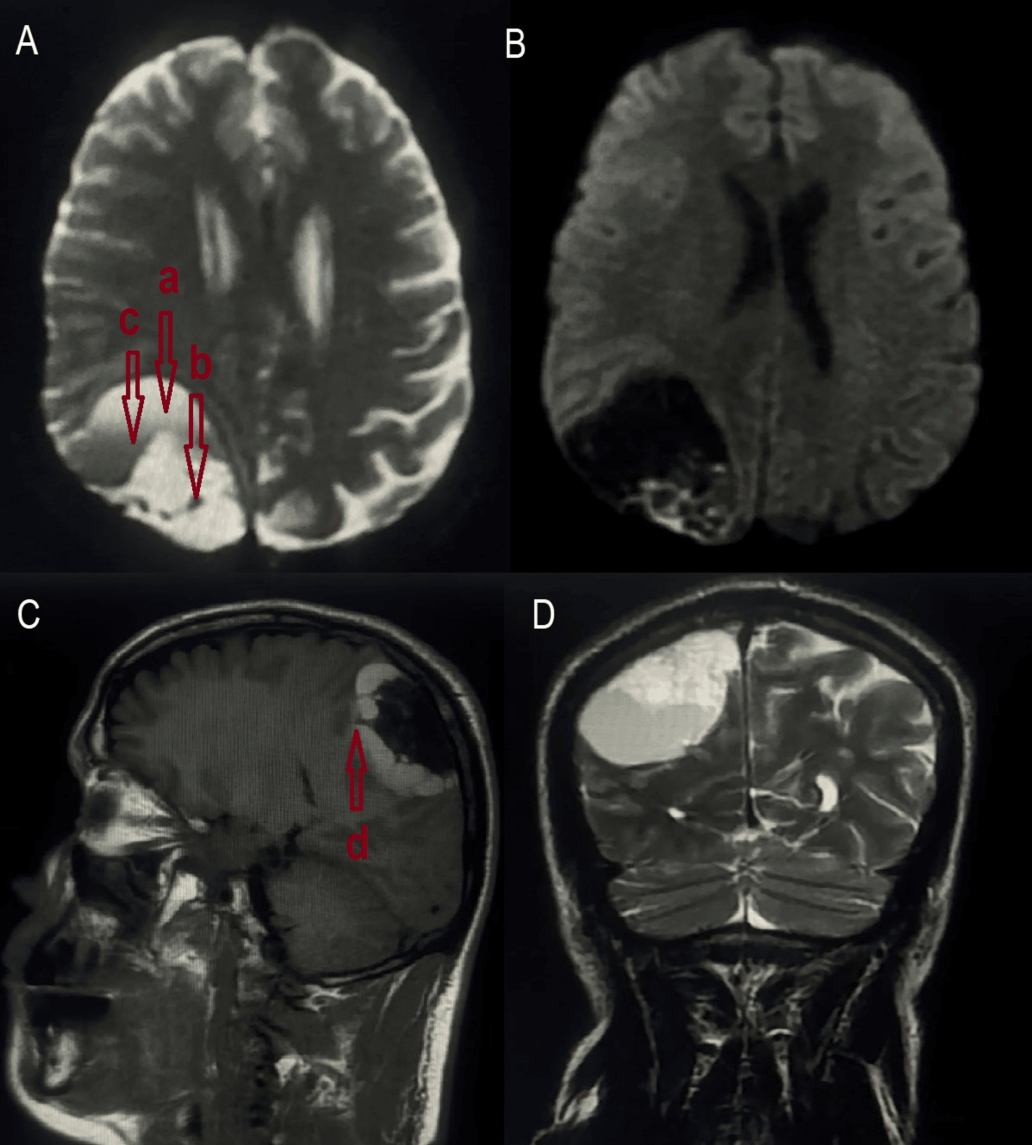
Brain MRI revealed a right parietal lesion with a broad dural attachment. It was predominantly cystic, featuring a posterior solid component exhibiting low signal intensity on T1-weighted images (A, C) and high signal intensity on T2-weighted images (D). The anterior cystic portion displayed hemorrhagic characteristics, with high signal intensities on T1, T2, and FLAIR sequences, alongside a fluid-fluid level indicative of rebleeding (A, D). a: Cystic portion of the lymphangioma; b: Solid portion of the lymphangioma; c: Fluid-fluid level indicating rebleeding; d: Septa partitioning the cystic part of the lymphangioma FLAIR: fluid-attenuated inversion recovery
